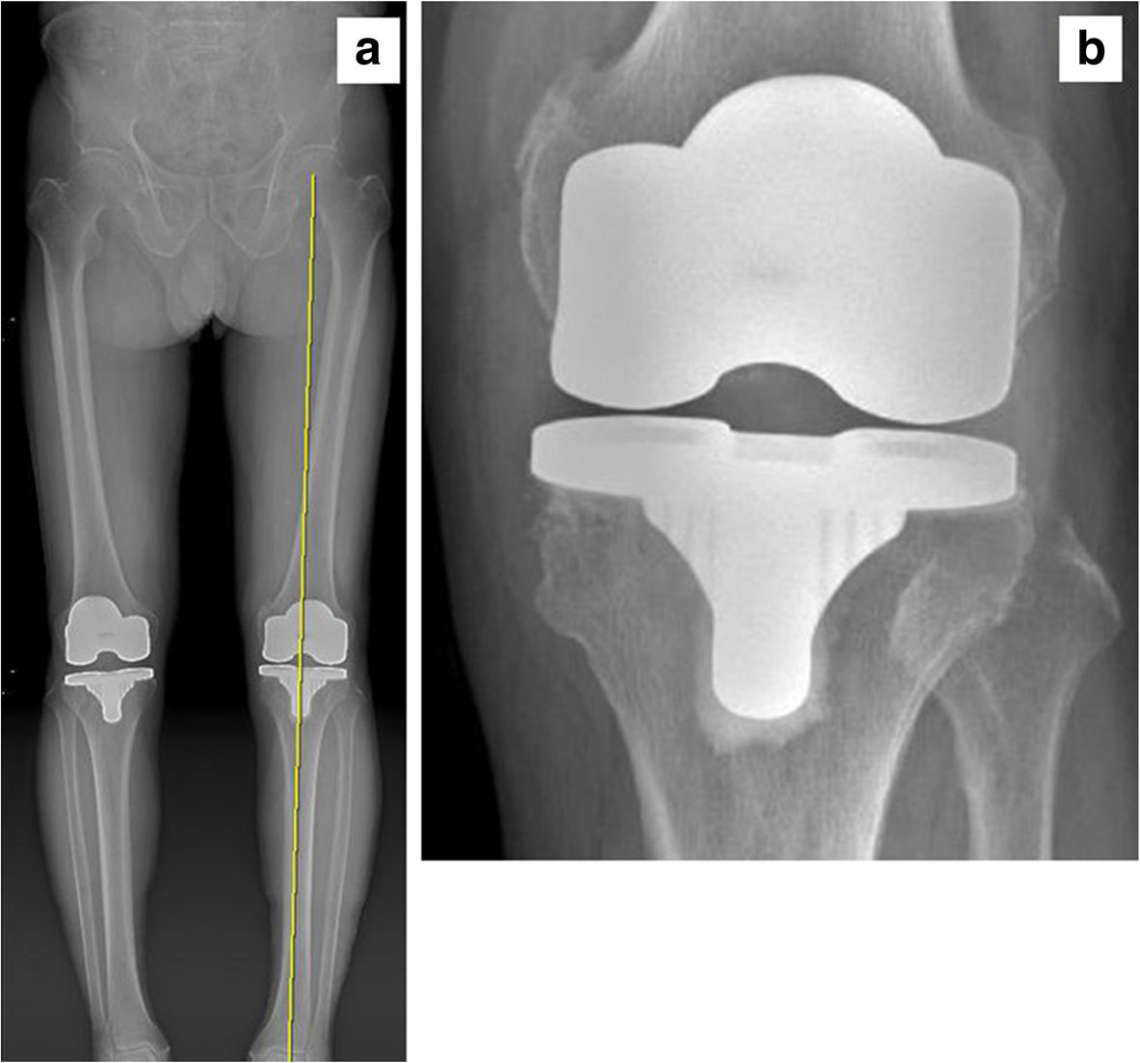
a Postoperative long-standing anteroposterior and (b) anteroposterior radiographs at year of an 72-year-old woman with the AOBG showed no migration of implants and presence of radiolucent lines at the bone cement-prosthesis interface
